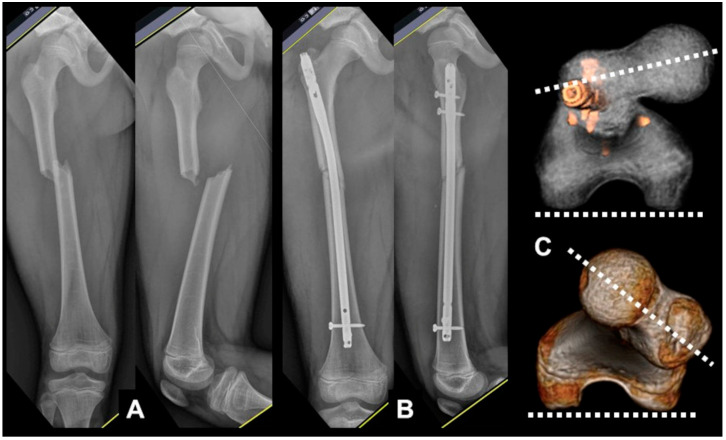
(A) An 11-year-old male patient diagnosed with a femoral-shaft fracture; (B) IM nailing was carried out; (C) postoperative CT scan showing the decreased angle of anteversion compared with the noninjured side.
